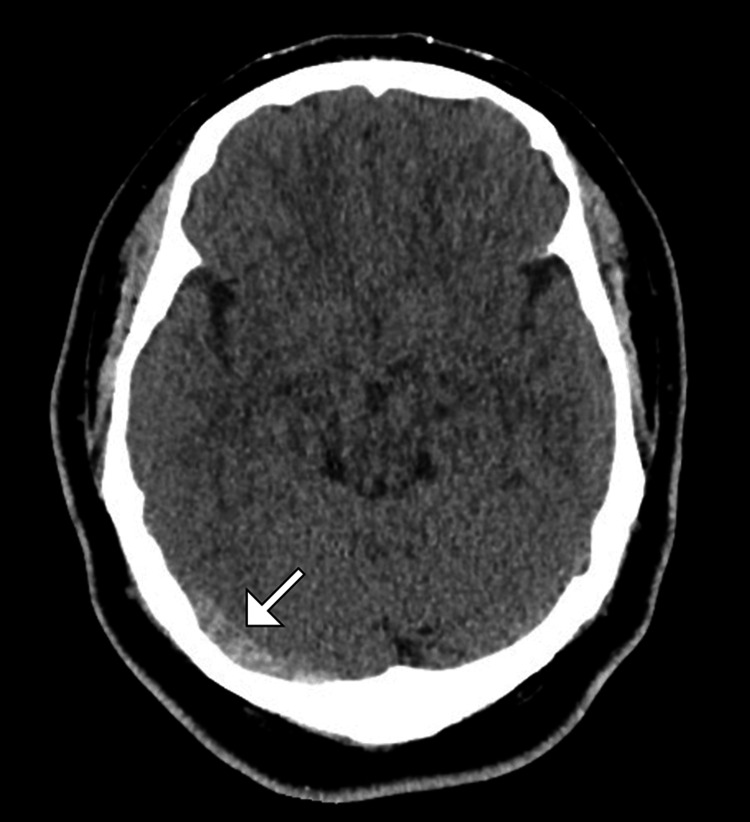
Axial non-contrast brain computed tomography image showing hyperdensity (arrow) along the transverse sinus
